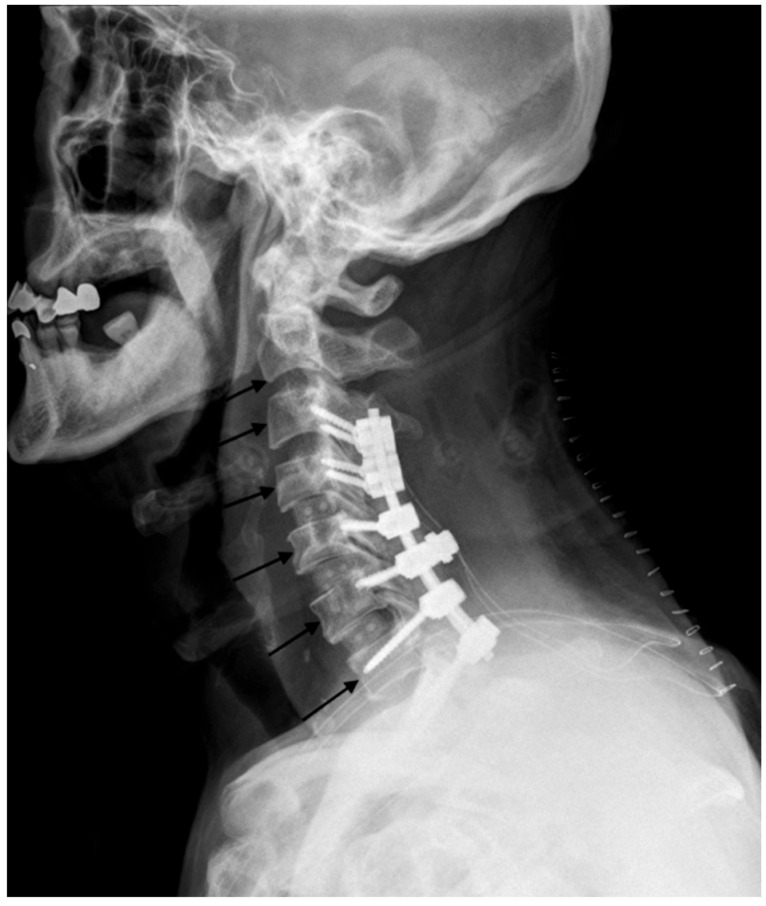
Plain radiograph showing PSTS measurements.
